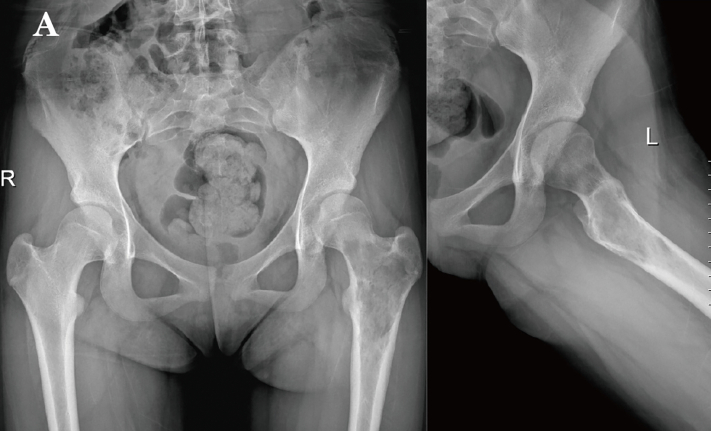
Preoperative X-ray revealed a cystic lesion in the proximal left femur.
